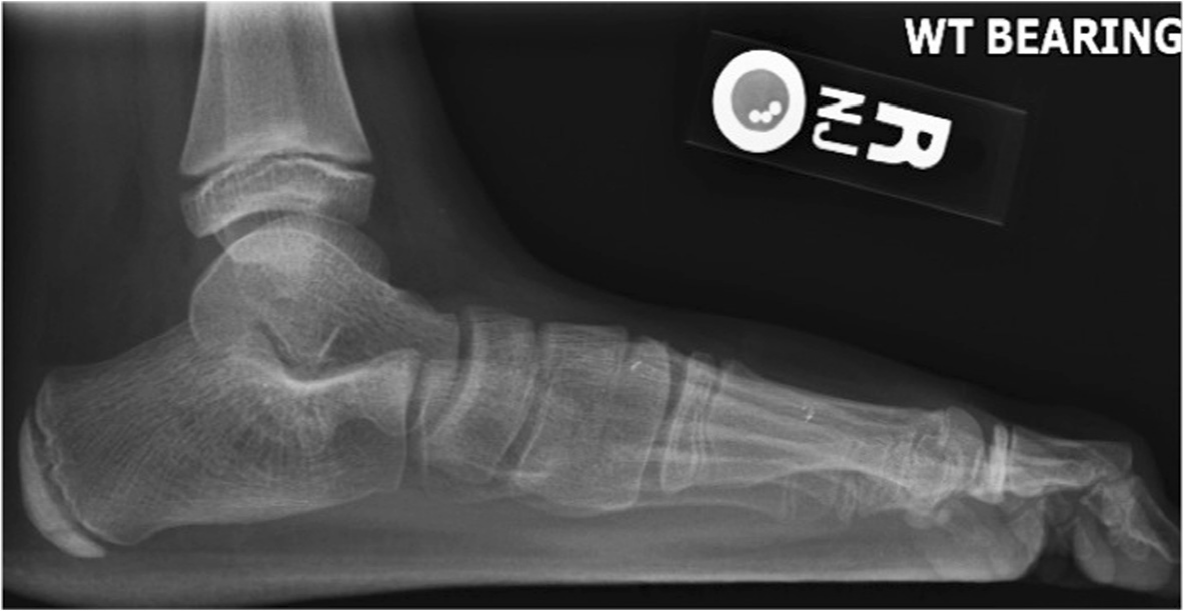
The lateral X-ray image shows the C sign.
